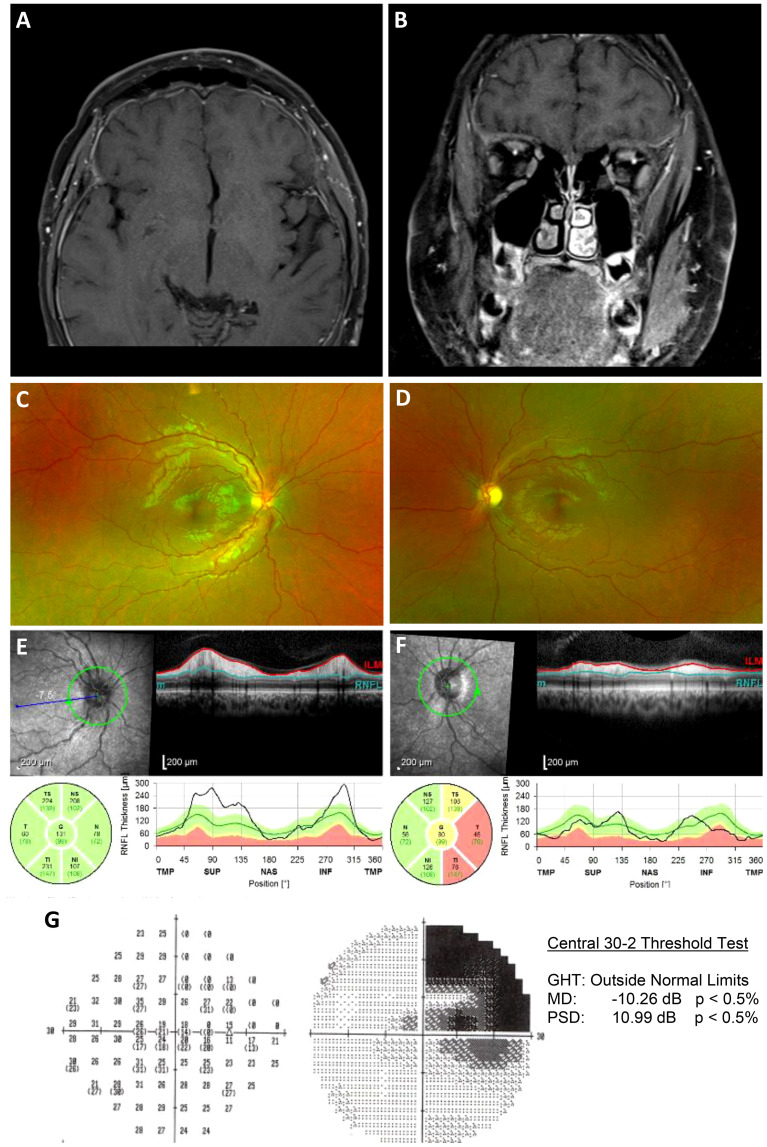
Follow-up post immunosuppressive treatment. (A, B), Axial and coronal T1-weighted postcontrast fat-saturated images after treatment with steroids and IVIG demonstrates a significant interval decrease in dural thickening, with only minimal residual enhancement. (C, D), Widefield fundus photographs demonstrating an improvement in optic nerve edema of both eyes, a marked temporal pallor of the left eye, and resolution of multifocal subretinal infiltrates along the superior and inferior temporal arcades of the left eye. (E, F), Circumpapillary RNFL thickening improved OD and demonstrated inferotemporal thinning OS. (G), Automated perimetry demonstrating an improvement in right superior quadrantanopia, which was previously thought to be due to a ganglioglioma resection.
